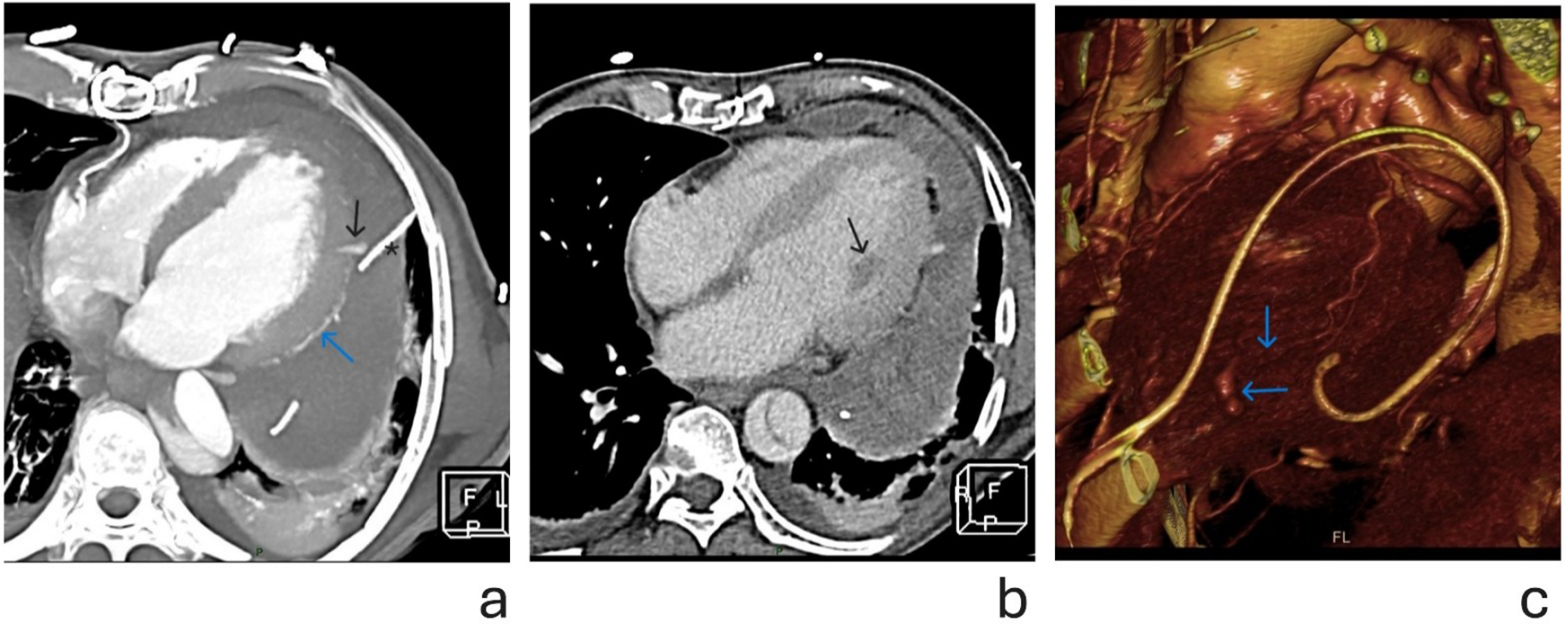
(a) Cross sectional thickened maximal intensity projections (MIP) image of cardiac CT after pericardiocentesis, in early arterial phase. Blue arrow shows the course of OM branch. Black arrow points to the contrast extravasation, at distal part of the OM branch. Note the proximity of the pericardial catheter (marked with *) to the site of hemorrhage. (b) Axial cross section CT image showing heterogeneous pericardial effusion in portovenous phase. Black arrow points to hypodense (ischemic) anterolateral papillary muscle, irrigated by OM branch, further suggesting its lesion. (c) Volume rendering (VR) reconstruction showing—blue arrows pointing to distal OM and site of contrast extravasation.
